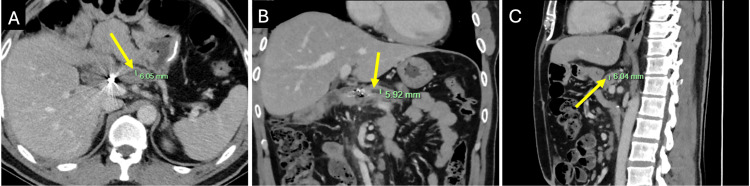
CT imaging in February 2025 demonstrated stable main pancreatic duct dilatation and no metastatic recurrence Portal venous phase contrast-enhanced CT images in (A) axial, (B) coronal, and (C) sagittal views demonstrating a 6 mm main pancreatic duct (arrow) on surveillance in February 2025. CT, computed tomography.
